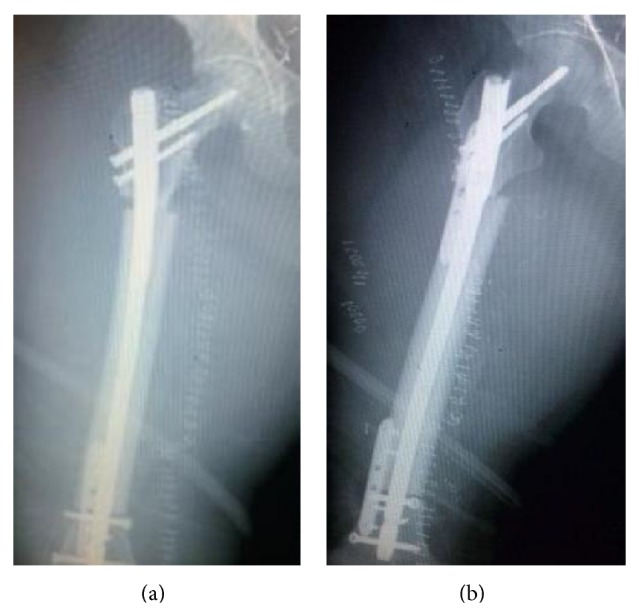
((a), (b)) Early postoperative AP and lateral X-rays.
